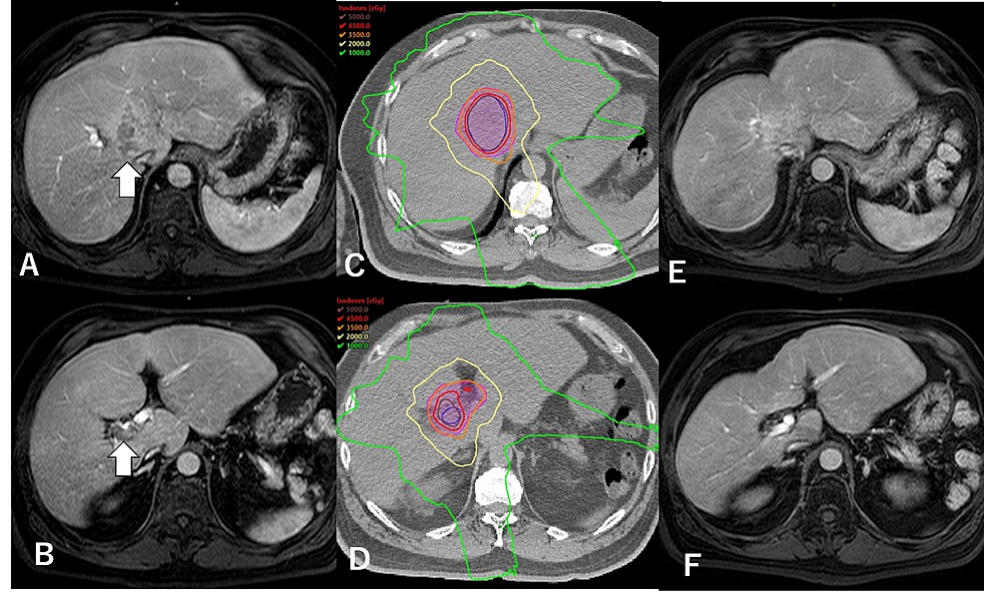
Case 1 – SBRT monotherapy A, B: pretreatment MRI of the liver demonstrating hepatocellular carcinoma invading bilateral portal vein invasion (arrows). C, D: axial images of the dose distribution of radiotherapy. SBRT was performed with 35 Gy in five fractions prescribed to enclose the planning target volume with a 60% isodose line of the maximal dose equated to the prescribed dose. E, F: the latest MRI with no recurrence 24 months after SBRT. The portal vein was kept flowing MRI: magnetic resonance imaging: SBRT: stereotactic body radiotherapy
